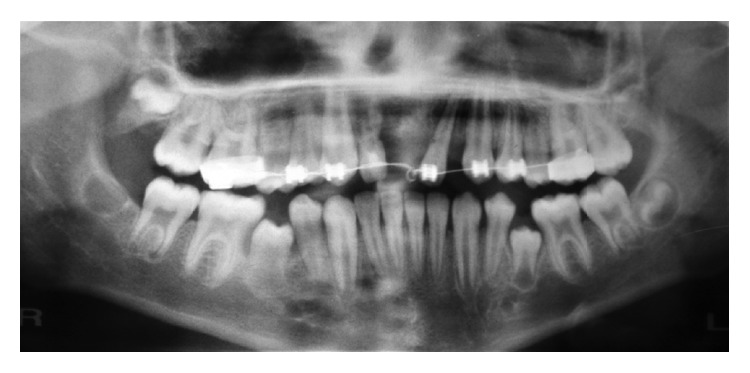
Radiographic view showing root resorption of maxillary lateral incisor.
